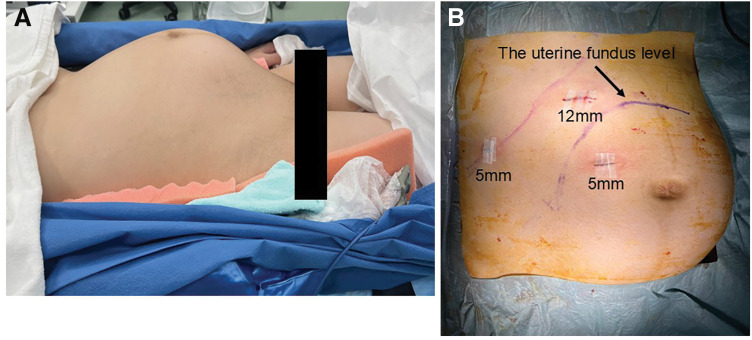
Intraoperative positioning and port placement in Case 5 (33 weeks of gestation). (A) A triangular pillow was placed under the right lumbar region to achieve a left lateral decubitus position; a positioning mat was used to facilitate intraoperative repositioning. Note: The positioning mat is not clearly visible in the photograph due to surgical draping, but was placed beneath the patient prior to the procedure to enable position adjustment if required. (B) The uterine fundus level was identified and marked on the abdominal surface based on preoperative CT. A 12-mm camera port was inserted in the right subcostal region using the open (Hasson) technique, and 2 additional 5-mm working ports were placed in a coaxial configuration.
